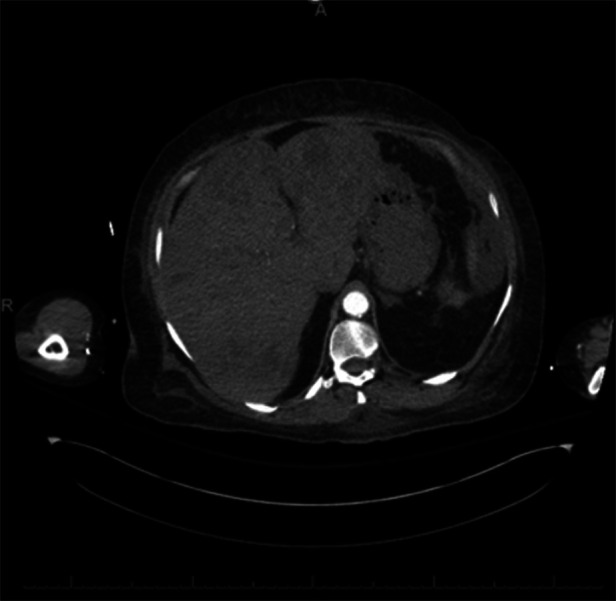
Abdominal computed tomography at time of admission showing cirrhotic liver morphology without any signs of laceration, hematoma, or malignancy.
